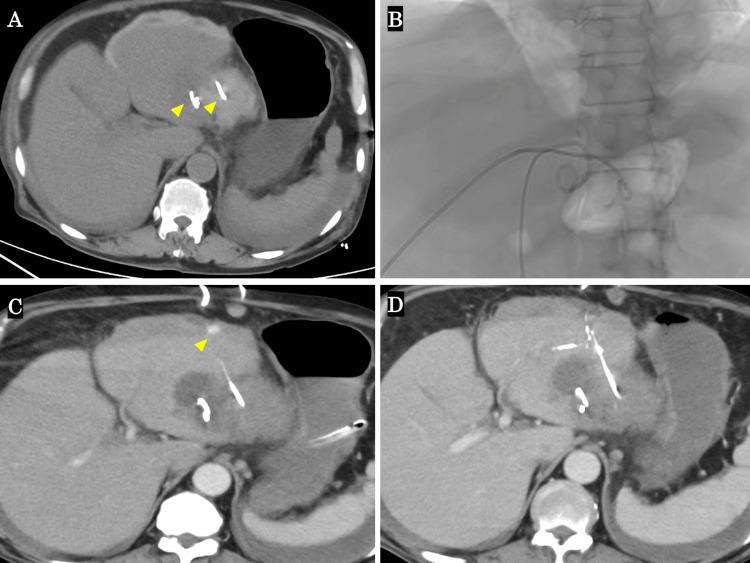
Plain CT and contrast-enhanced CT findings. (A, B) Liver abscess drainage was performed using a combination of ultrasound guidance and fluoroscopy (yellow arrows). (C) Extravasation of the contrast medium was observed on the surface of the liver (yellow arrow). (D) TAE was performed on the hepatic artery A3. CT: computed tomography, TAE: transcatheter arterial embolization
